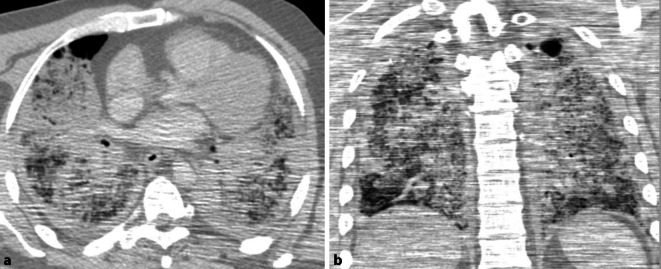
CT scans of the lungs in axial (a) and coronary (b) projections with bilateral pleural effusions, global multifocal reticular consolidation and subpleural milky glass opacities
